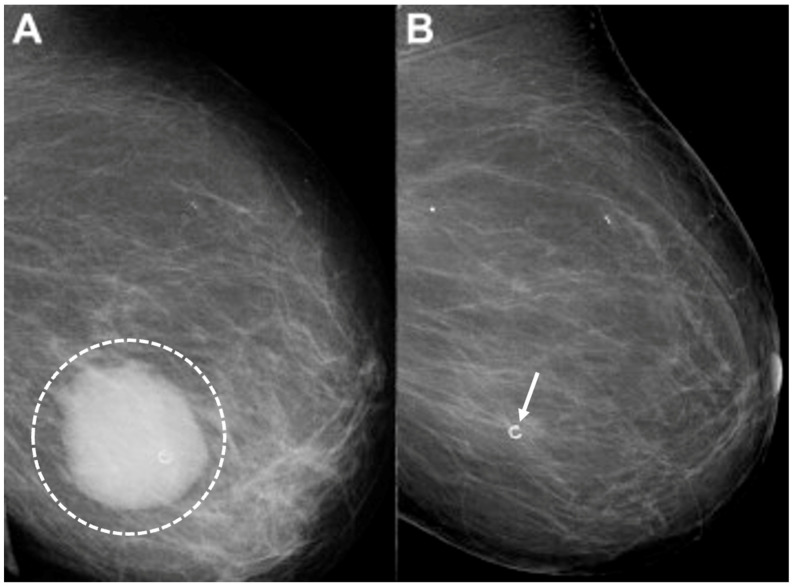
Mammogram performed before (A) pre-operative chemotherapy showing large, white, ultrasound visible cancer encircled by hash marks. After completing pre-operative chemotherapy, a repeat mammogram (B) of the same breast showed complete disappearance of the original cancer leaving only a circular metal biopsy site marker (arrow) to indicate the location of the original cancer.
